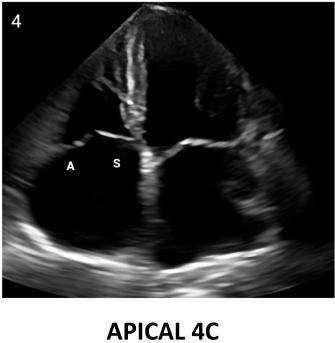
In the apical 4C view (Box 4) of the RV, S is clearly identifiable by using the interventricular septum as landmark. The opposing leaflet is A in 81% of cases. Tilting the probe anteriorly to image AV allows for the clear visualization of A and S. Conversely, P and S become visible with posterior tilting (along with the CS).
